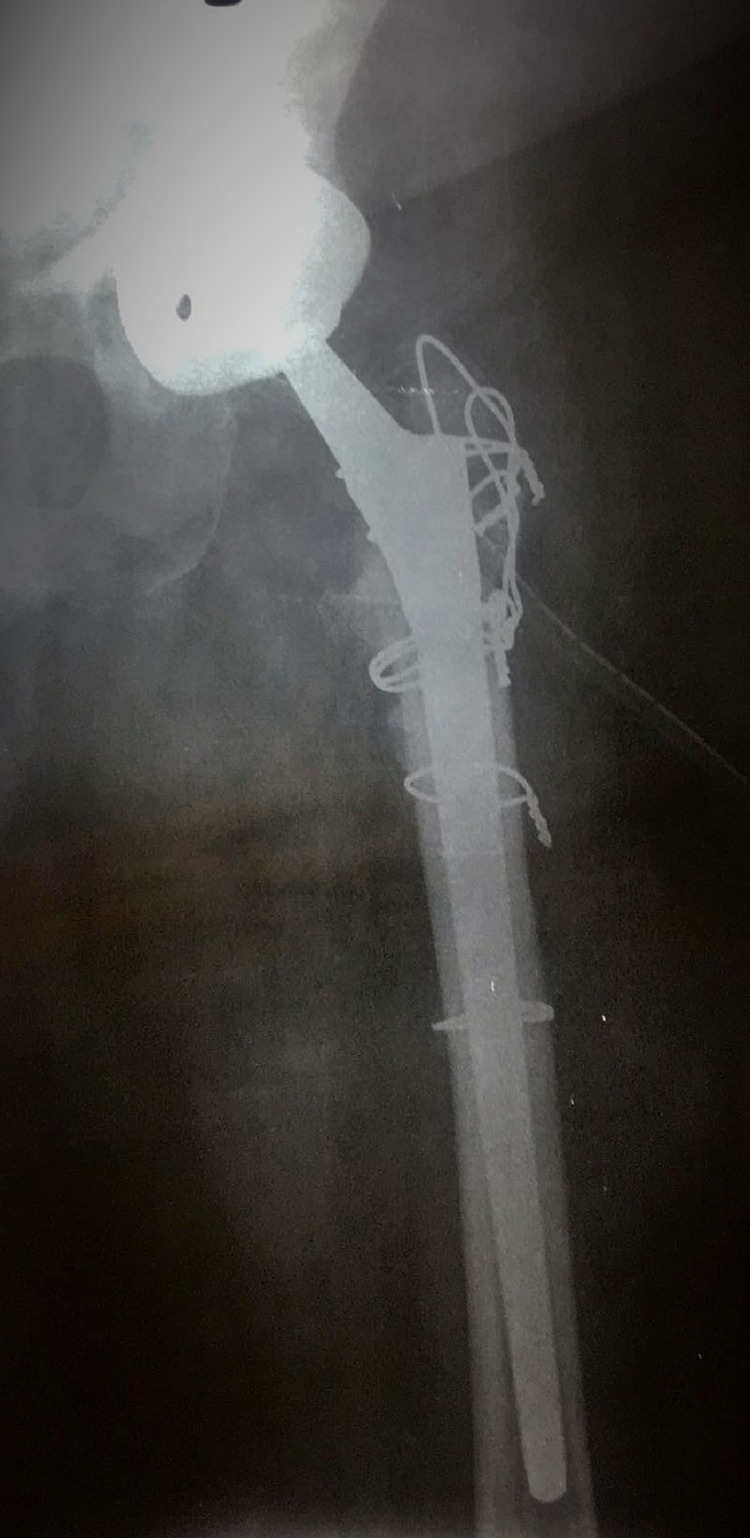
Patient’s postoperative X-ray
